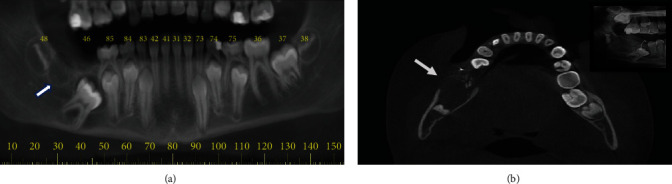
(a) Panoramic reconstruction of an initial CBCT showing a hypodense image (white arrow) in the region of a second molar suggesting the presence of a dental germ. (b) CBCT axial view showing the lesion expansion and cortical fenestration (white arrow). Foci of peripheral calcification in the lesion (in box).
